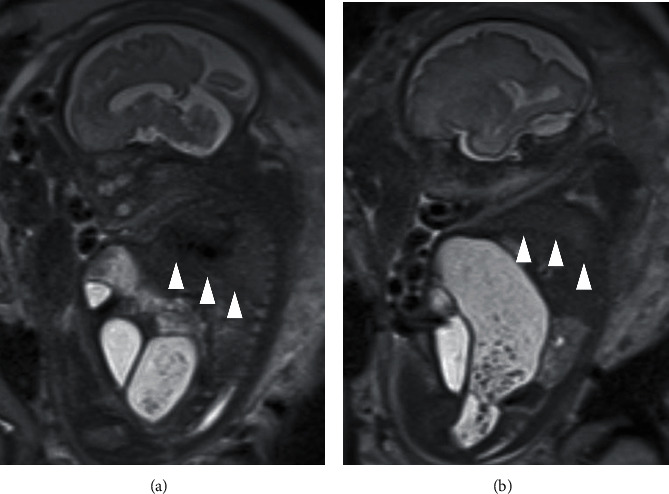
Fetal MRI. Fetal T2-weighted magnetic resonance imaging at 30 weeks showing small lungs with bowel dilation and oligohydramnios. The arrowheads indicate the diaphragm.
